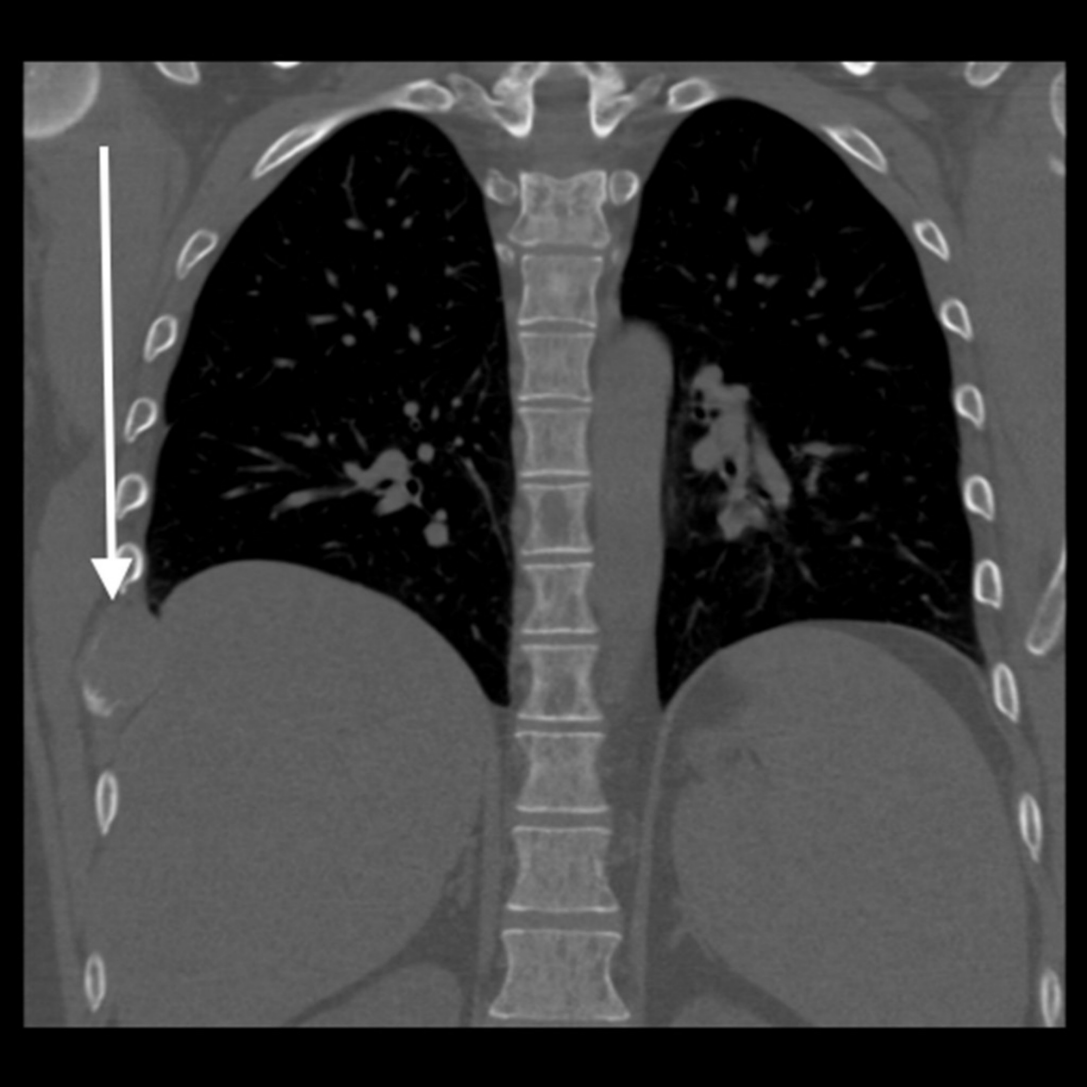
Coronal CT image on bone windows shows the lyric expansive rib lesion, corresponding to the chest x-ray abnormality. No fracture was seen. Pleura was normal. CT: computed tomography
